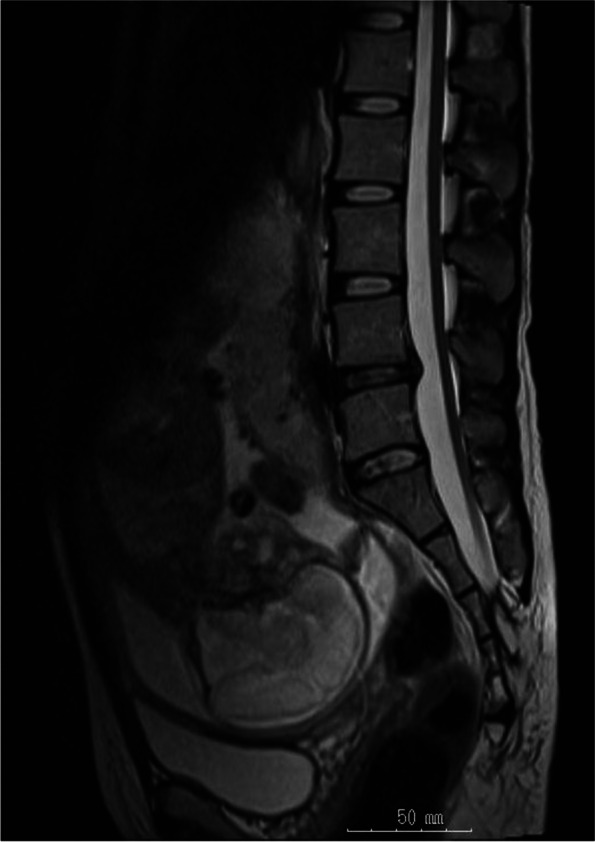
MRI scan of the lumbosacral region. The spinal cord is elongated and tethered to the sacral subdermal lipoma. Normal epidural space is preserved in the lumbar region
